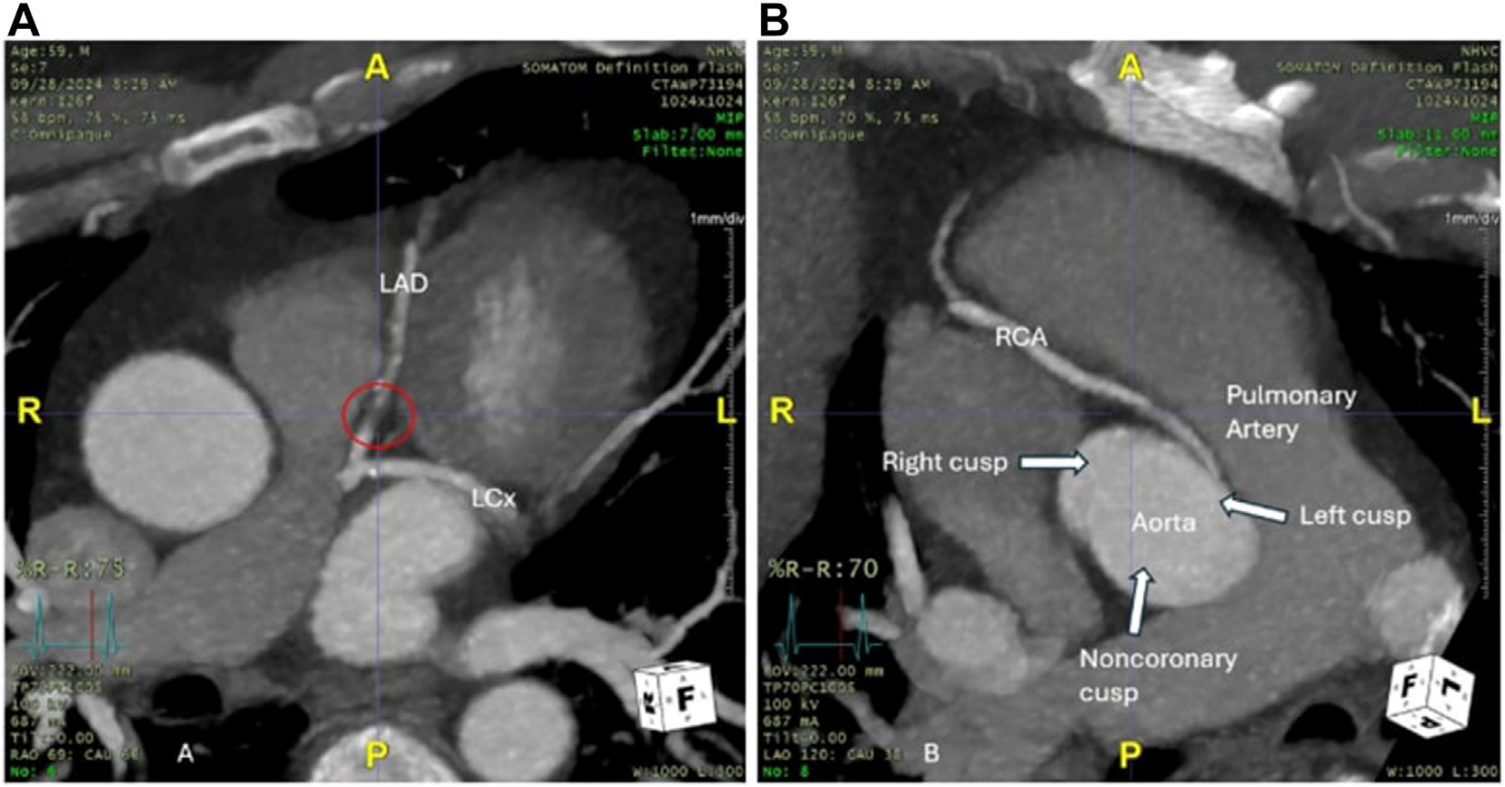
Outpatient coronary computed tomography angiography images. (A) The soft, microcalcified plaque in the left anterior descending coronary artery (LAD) is circled. (B) An anomalous right coronary artery (RCA) can be seen arising from the left cusp. Its course is intramural (attached to the cardiac muscle) and interarterial (between the aorta and the pulmonary artery). LCx, left circumflex artery.
